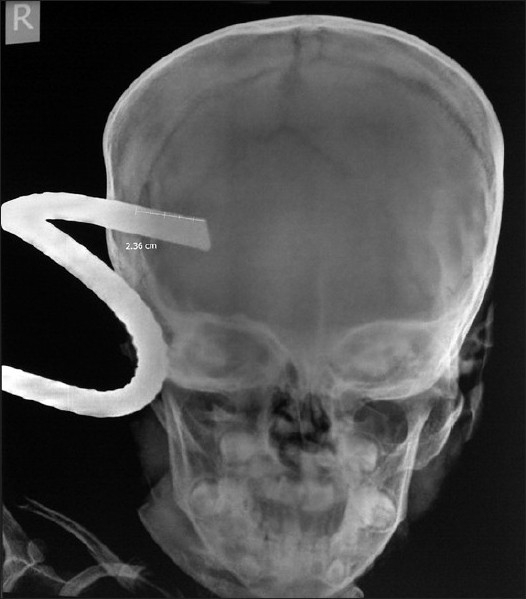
X-ray skull showing iron rod penetrating inside
